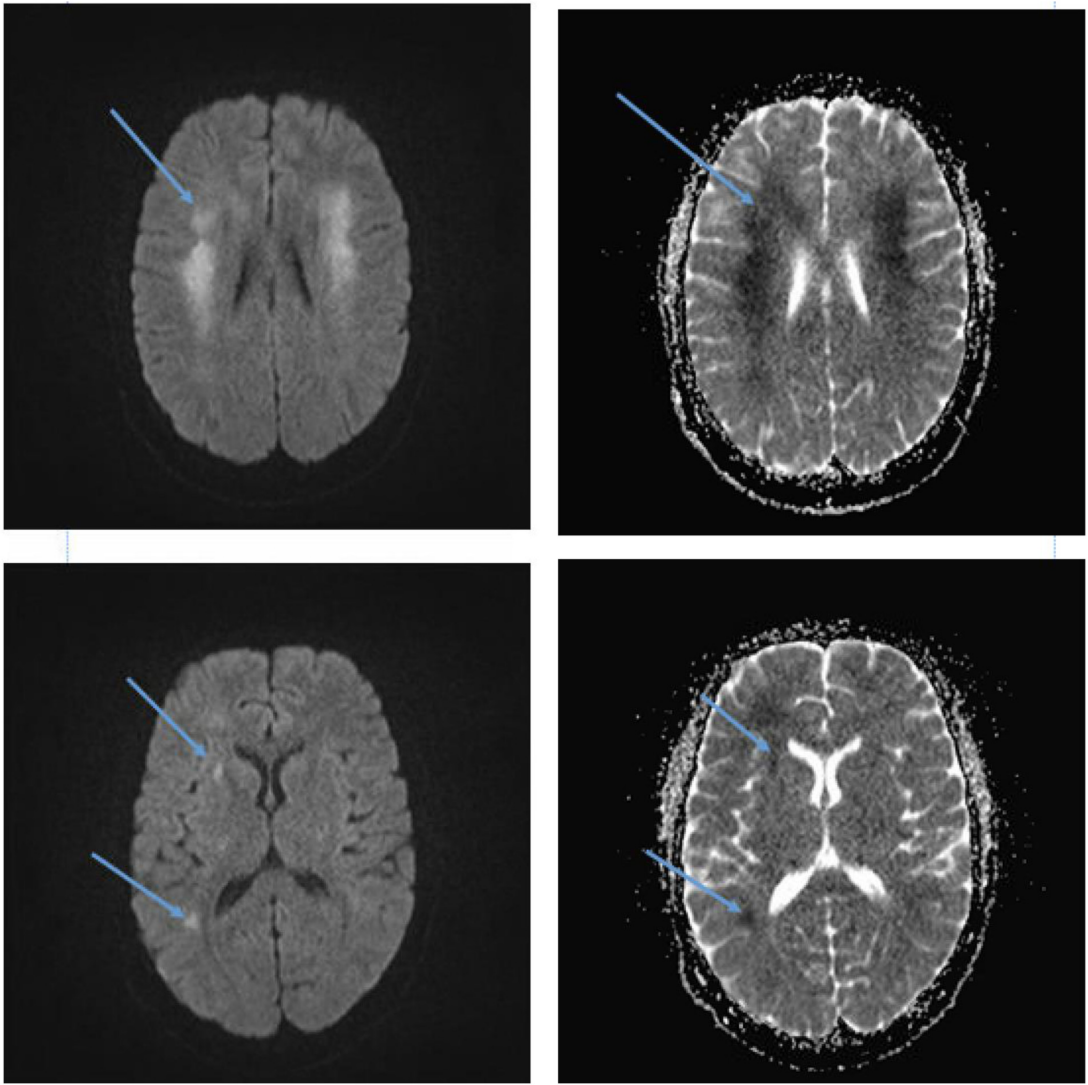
MRI brain DWI-ADC showing restricted diffusion involving the bilateral corona radiata (top images) and small areas of restricted diffusion involving the right anterior limb of the internal capsule and the right occipital white matter (bottom images).
